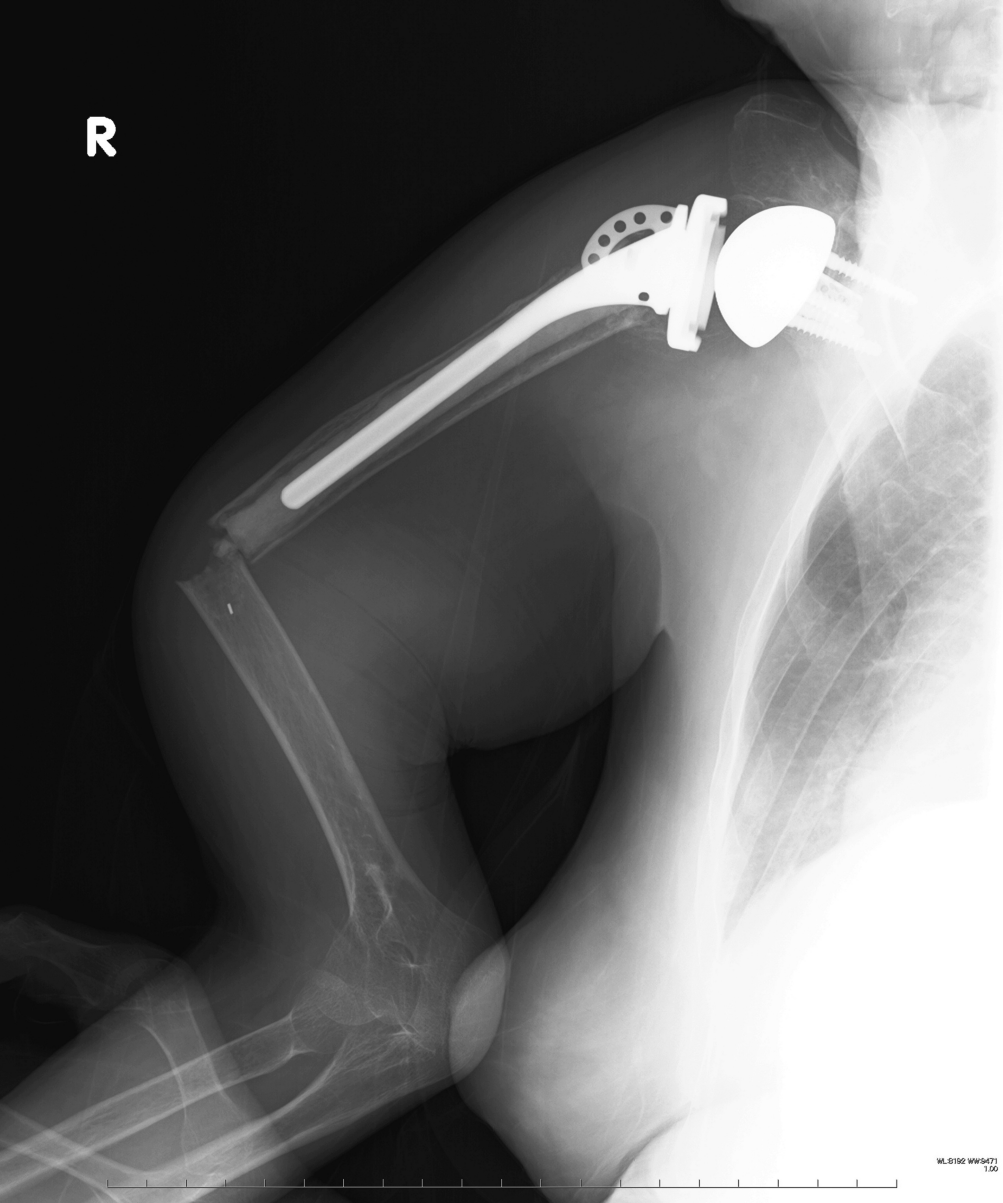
Anteroposterior plain radiograph at eight months after reverse shoulder arthroplasty A periprosthetic fracture is observed at the distal tip of the cement.
